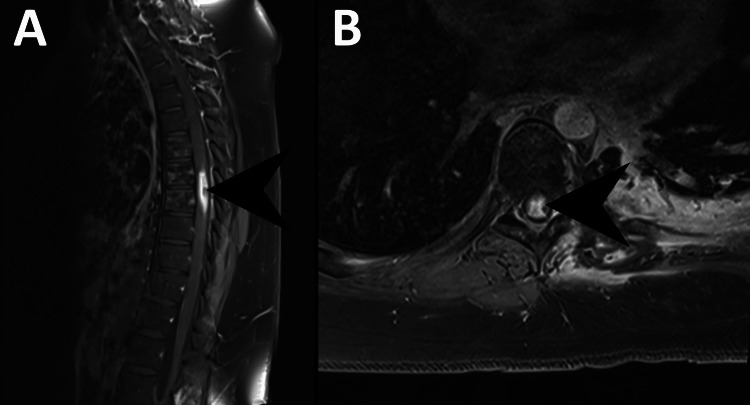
MRI A: Post-contrast MRI T1 sagittal view of the thoracic spine, demonstrating cord enhancement extending from T7-T8, most suggestive of radiation necrosis (black arrowhead). B: Post-contrast MRI T1 axial view of the thoracic spine, re-demonstrating cord enhancement (black arrowhead)
